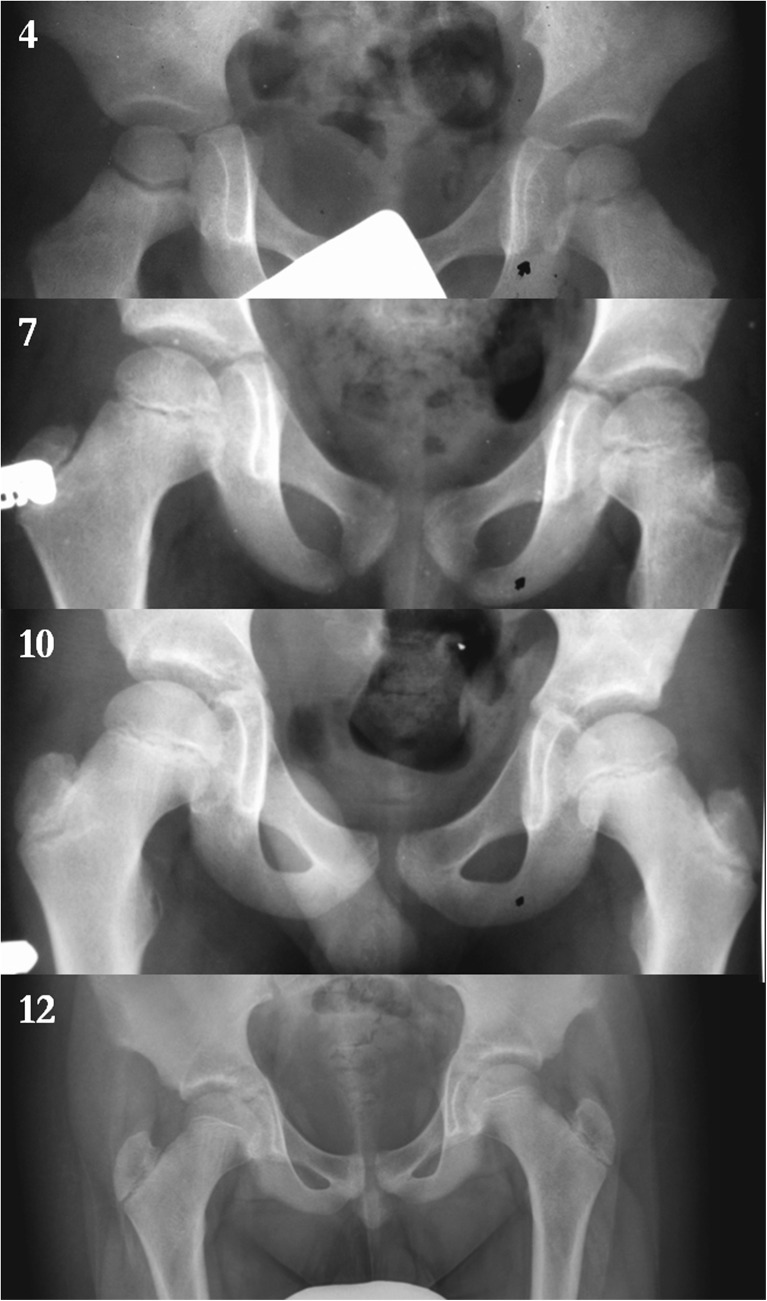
X-rays showing evolution of the growth plate of the proximal femoral epiphysis in a 4-, 7-, 10- and 12-year-old child
